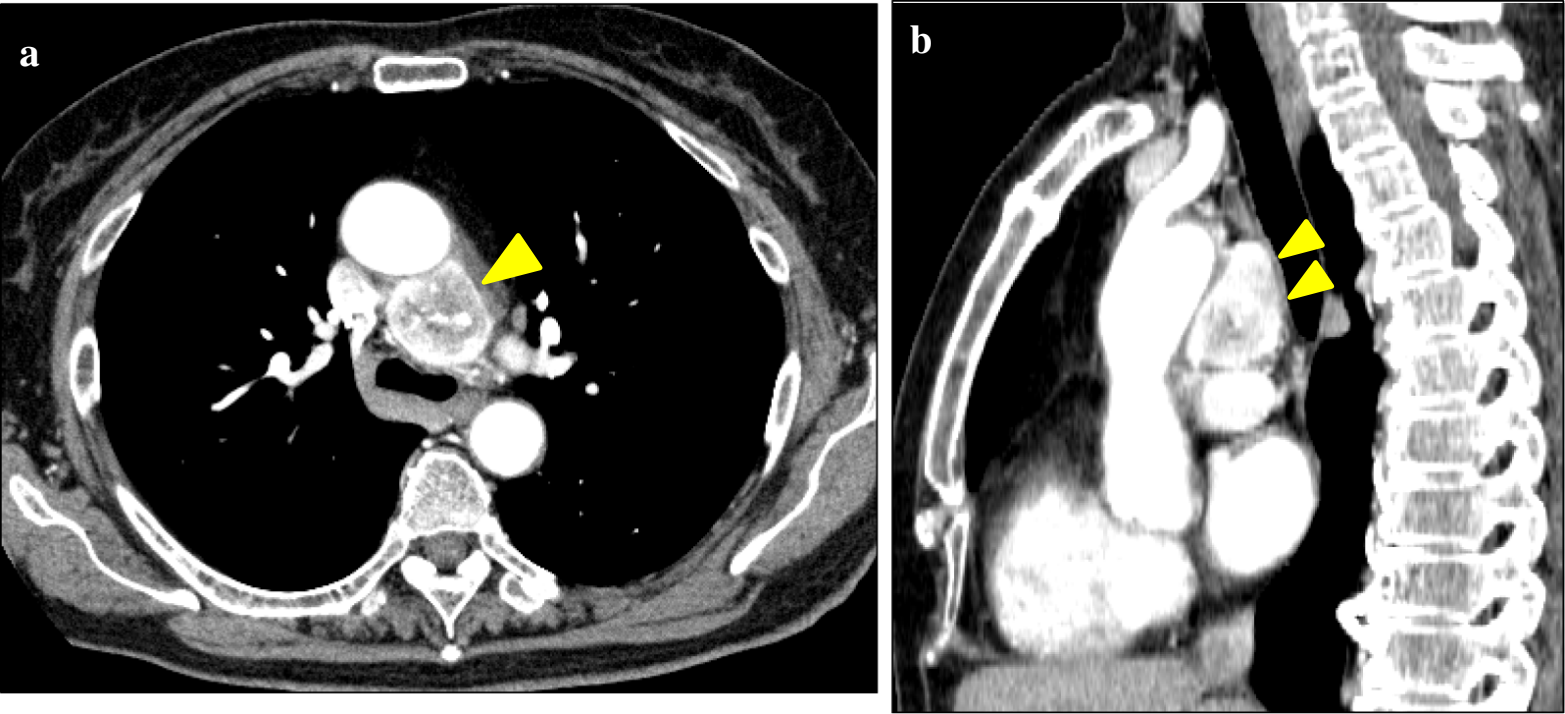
Chest CT scan. Preoperative contrast-enhanced chest computed tomography scan showing a ring-enhancing tumor in the middle mediastinum (allow)
